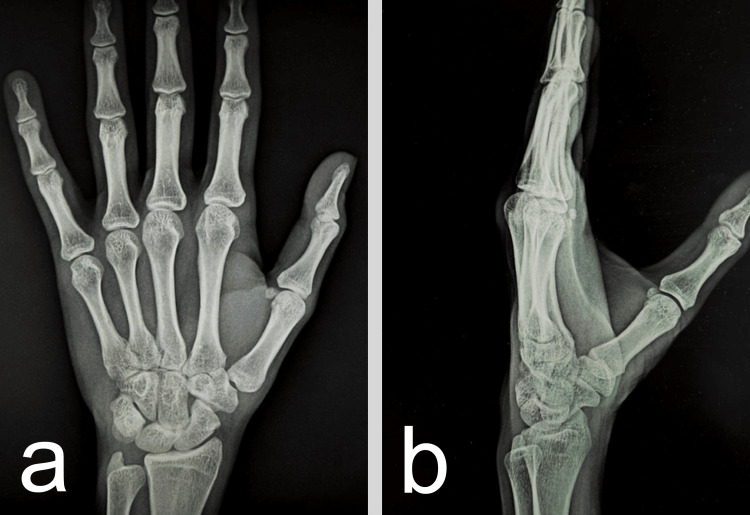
Follow-up anteroposterior (a) and lateral (b) radiographs showing no signs of subluxation or joint space narrowing
